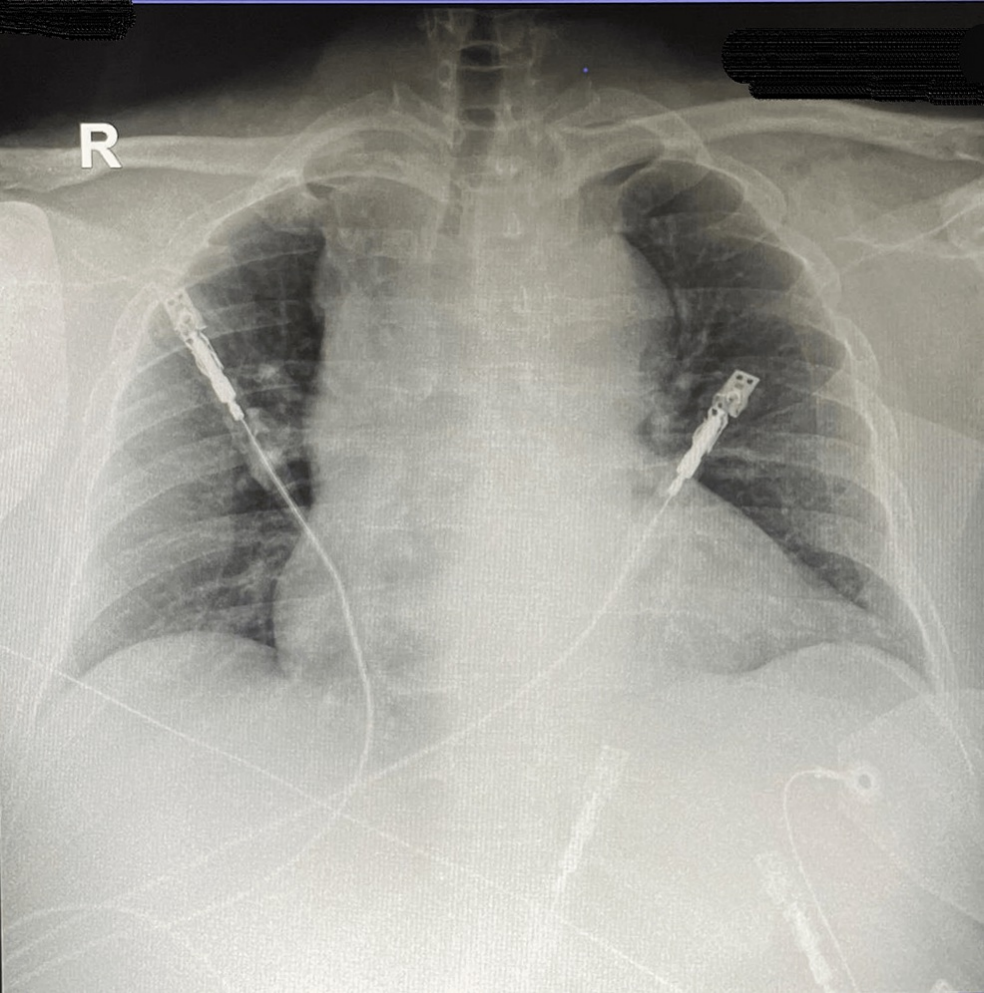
Portable chest X-ray showing generalized cardiomegaly with mediastinal widening
